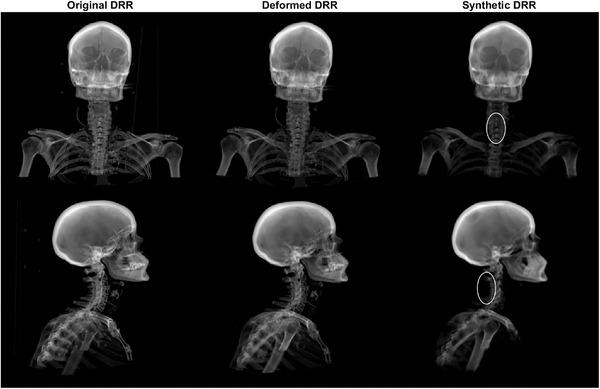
Example of frontal (top) and sagittal (bottom) original digital reconstructed radiograph (DRR) (left), deformed DRR (middle), and synthetic DRR (right). The ellipses show bone structures used for patient positioning that have somewhat decreased contrast and blurred edges in the synthetic DRR.
